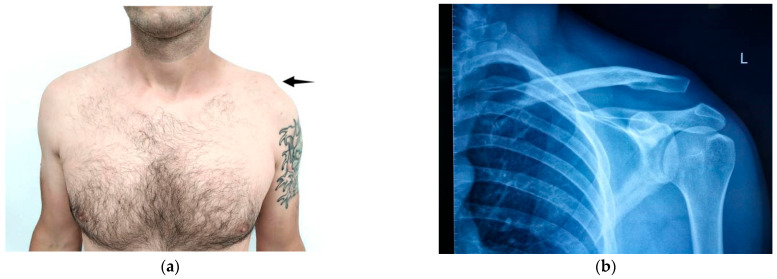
(a) Clinical aspect and (b) X-ray image of left AC separation.
